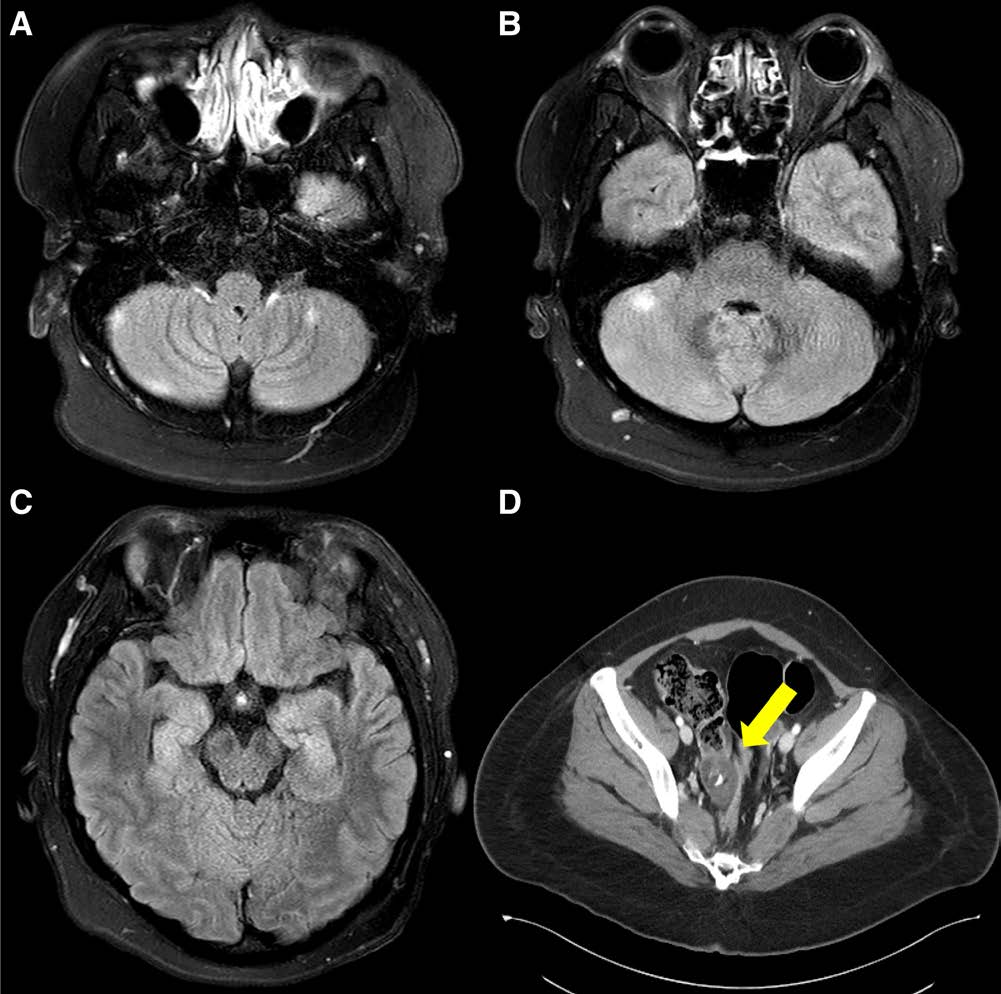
Brain magnetic resonance images (A–C) and enhanced abdominopelvic computed tomography (CT) image of the patient (D). Enhanced fluid-attenuated inversion recovery (FLAIR) images show enhancing lesions in bilateral cerebellum (A, B) and hippocampus (C). CT shows right ovarian mass with calcification suggestive of teratoma (D, arrow).
